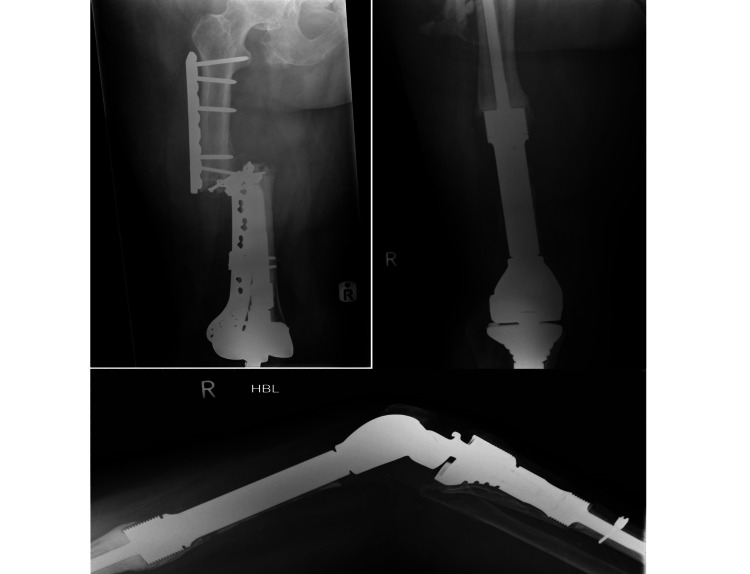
Radiographs for the same patient showing when the plate fixation failed due to non-union and was subsequently revised to a distal femoral replacement
